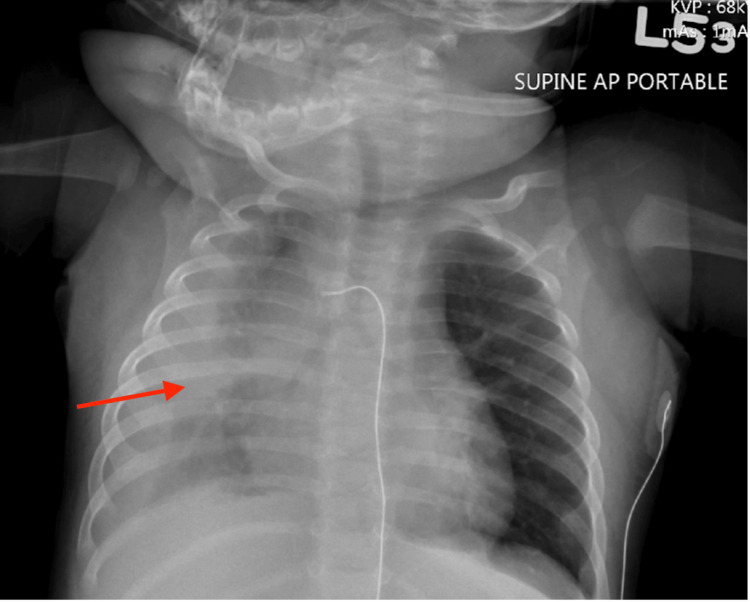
Chest X-ray with right-sided pleural effusion The initial chest X-ray of the patient when he presented with signs of respiratory distress. It shows signs of right lung infiltrate with a small, right-sided pleural effusion (arrow) AP: anteroposterior
